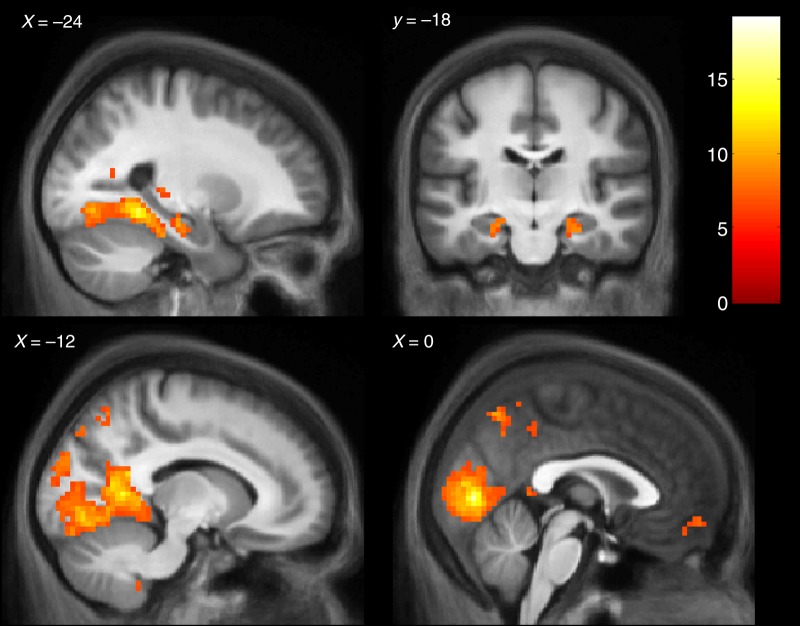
Main effect of scenes. Top-left: sagittal slice displaying left anterior medial hippocampus and PHC. Top-right: Coronal slice displaying anterior medial hippocampus. Bottom-left: Sagittal slice displaying left RSC and medial occipital cortex. Bottom-right: Sagittal slice displaying ventromedial prefrontal cortex, precuneus and medial occipital cortex. Calculated using the contrast of scene conditions relative to their individual object baselines ([perceive scenes + construct scenes + maintain perceived scenes + maintain constructed scenes] − [perceive objects + construct objects + maintain perceived objects + maintain constructed objects]). Thresholded at *P* < 0.05 FWE-corrected and displayed on the averaged structural MRI scan of the 25 subjects. The color bar indicates the *t*-statistic. See Table 1 for a full description of the results.
